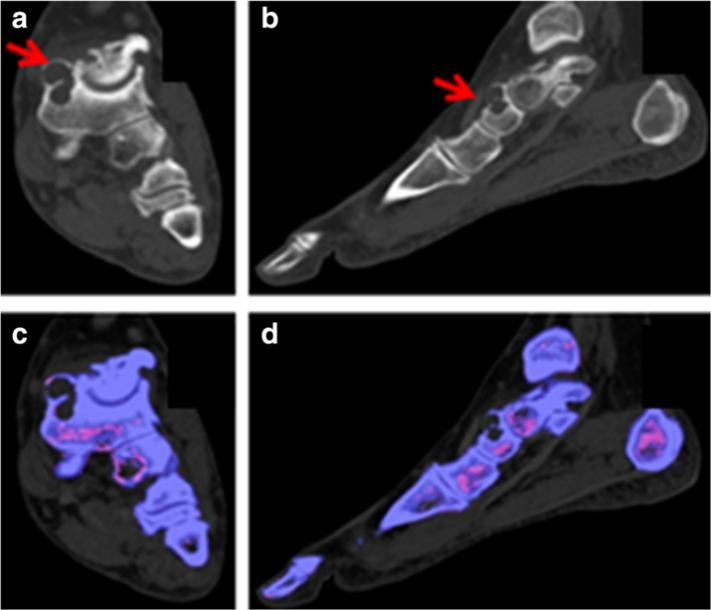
Patient 15. There is a large erosion of the dorsal navicular bone, seen on the coronal and sagittal CT images (panels (a) and (b), red arrows) but no corresponding green-coded (MSU) deposits in or around the erosion on the DECT images (panels (c-d))
